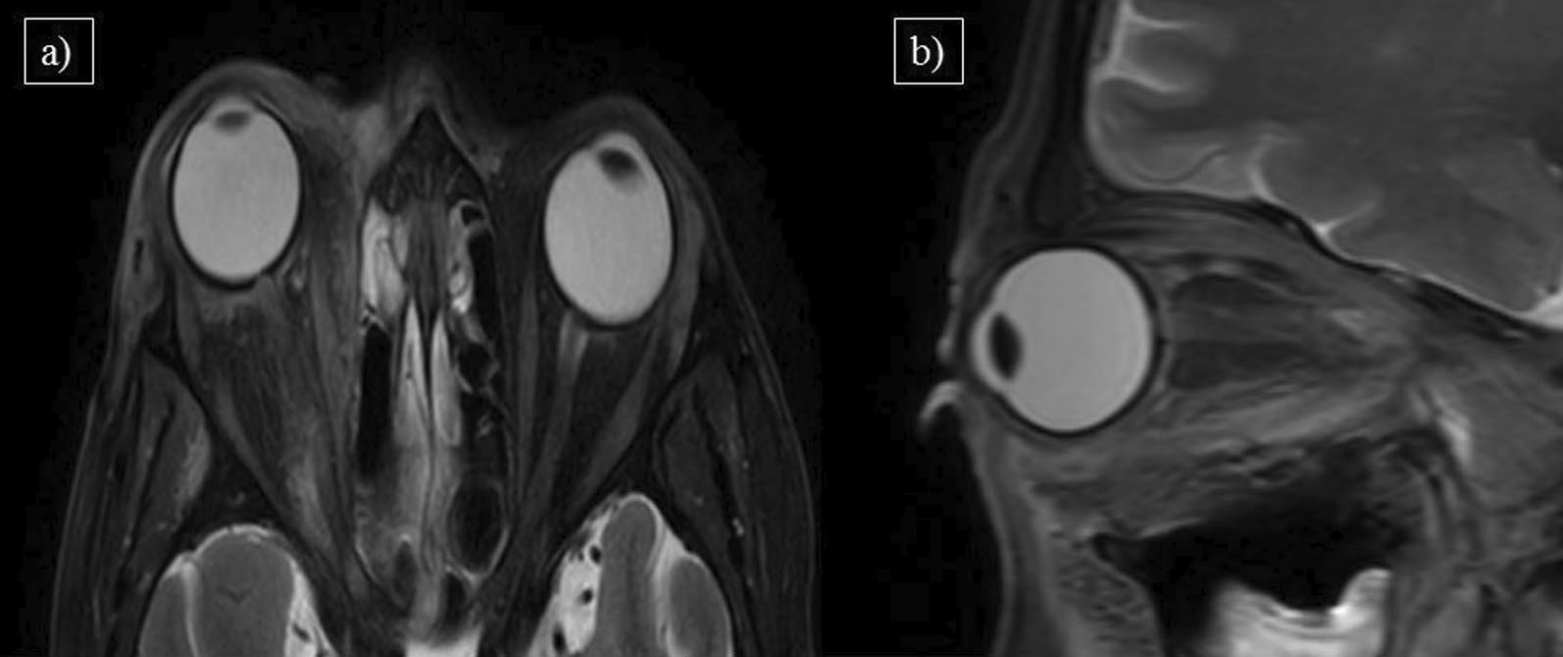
a MRI Axial T2W-FS and b sagittal T2W-FS images of a 50-year-old male patient with complaints of right sided proptosis and ocular pain showing features of pre and post-septal orbital cellulitis with resultant proptosis
